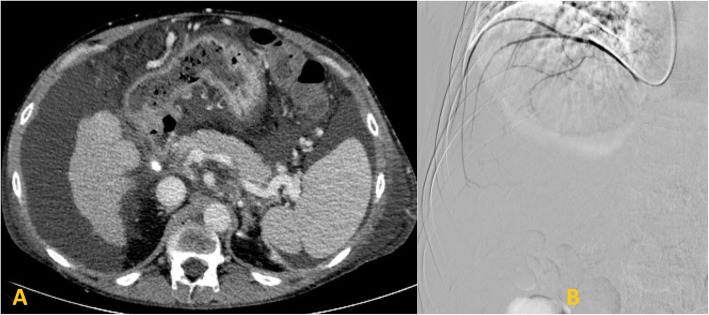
a: CT abdomen with contrast demonstrating occlusion of the extra-hepatic PV with cirrhotic morphology of liver, ascites and gastric varices. b: Venogram demonstrating non-opacification of any hepatic veins. Only a right inferior phrenic vein was selected and opacified
